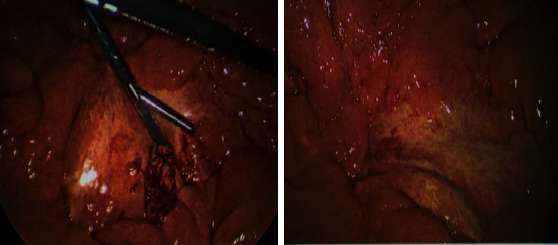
Mosaic white stranding of the mesentery in the proximal aspect in the areas involved on CT imaging. The bowel was hyperemic but obviously viable.
